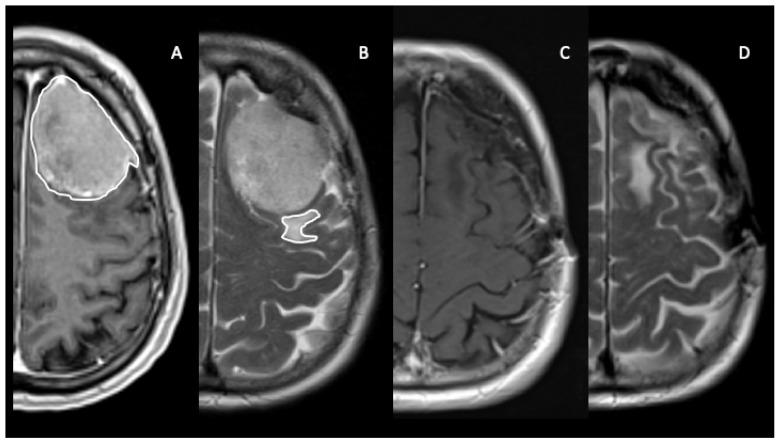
A brain MRI of a patient with left frontal meningioma. (A,B) show pre-operative postcontrast T1-weighted (A) and T2-weighted TSE MRI sequences, demonstrating ROI segmentation of lesion and peritumor edema, circumferentially outlined, using Horos software. (C,D) display postcontrast T1-weighted and T2-weighted TSE MRI sequences after surgical resection.
